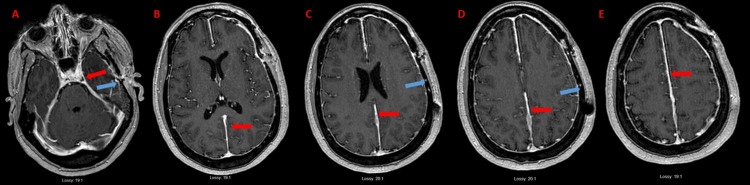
Follow-up MRI post-debulking surgery and RT. MRI post-T1 axial series show (A) enhancing tumor appears to have resolved at the sella, cavernous sinus, L optic nerve, and tentorium, (B-D) along the falx with no significant residual disease (red arrows), post-surgical changes (blue arrow). RT: radiation therapy; L: left
